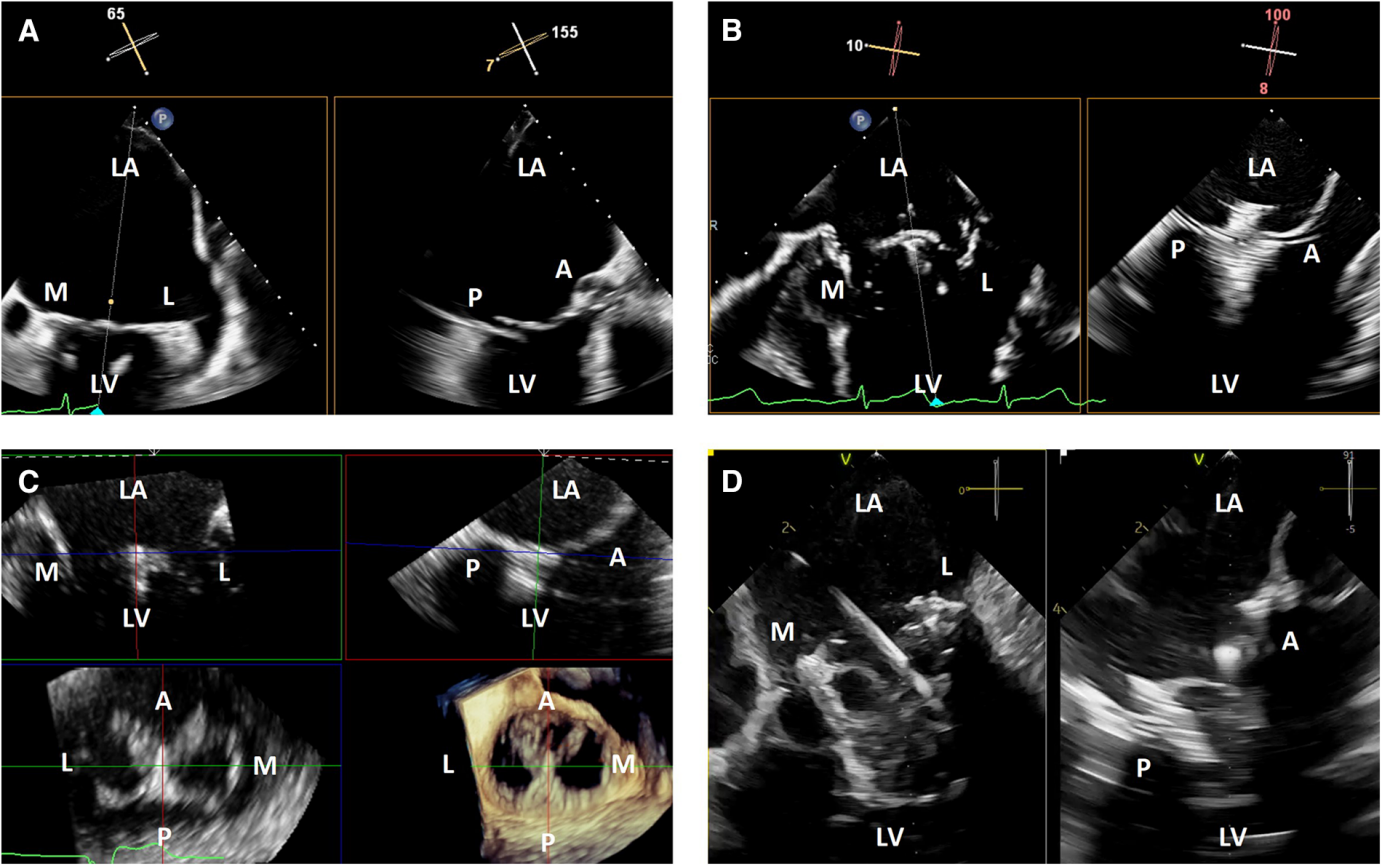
3-dimensional intracardiac echocardiography for transcatheter mitral interventions. (A) Biplane transesophageal echocardiography in a severely dilated left atrium causing reduced spatial resolution of the mitral valve due to its far field position; (B) biplane intracardiac echocardiography in the same patient with improved visualization of the mitral valve, allowing for a successful mitral transcatheter edge-to-edge repair; (C) live 3-dimensional multi-planar reconstruction from an intracardiac echocardiography volume after deployment of a mitral transcatheter edge-to-edge repair device; (D) biplane intracardiac echocardiography during a transcatheter mitral valve-in-valve implantation. LA, left atrium; M, medial; L, lateral; LV, left ventricle; A, anterior; P, posterior.
